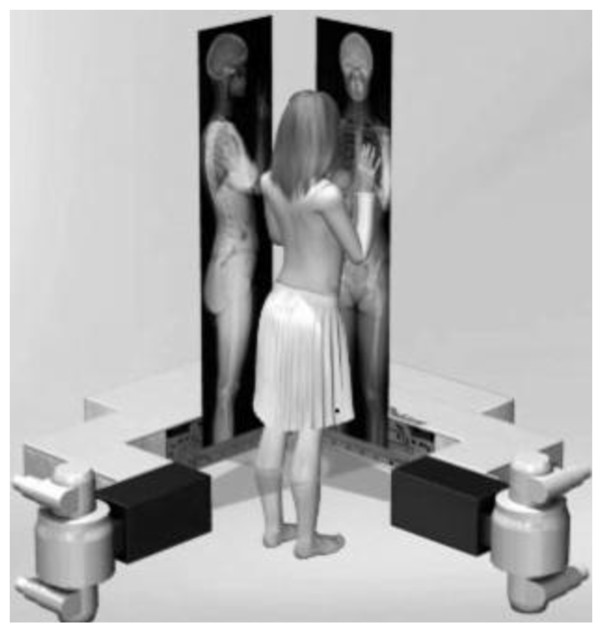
Images obtained by the ultra-low dose 2D/3D digital X-ray system used in this study. The system can provide total body X-rays for AP and lateral planes simultaneously
